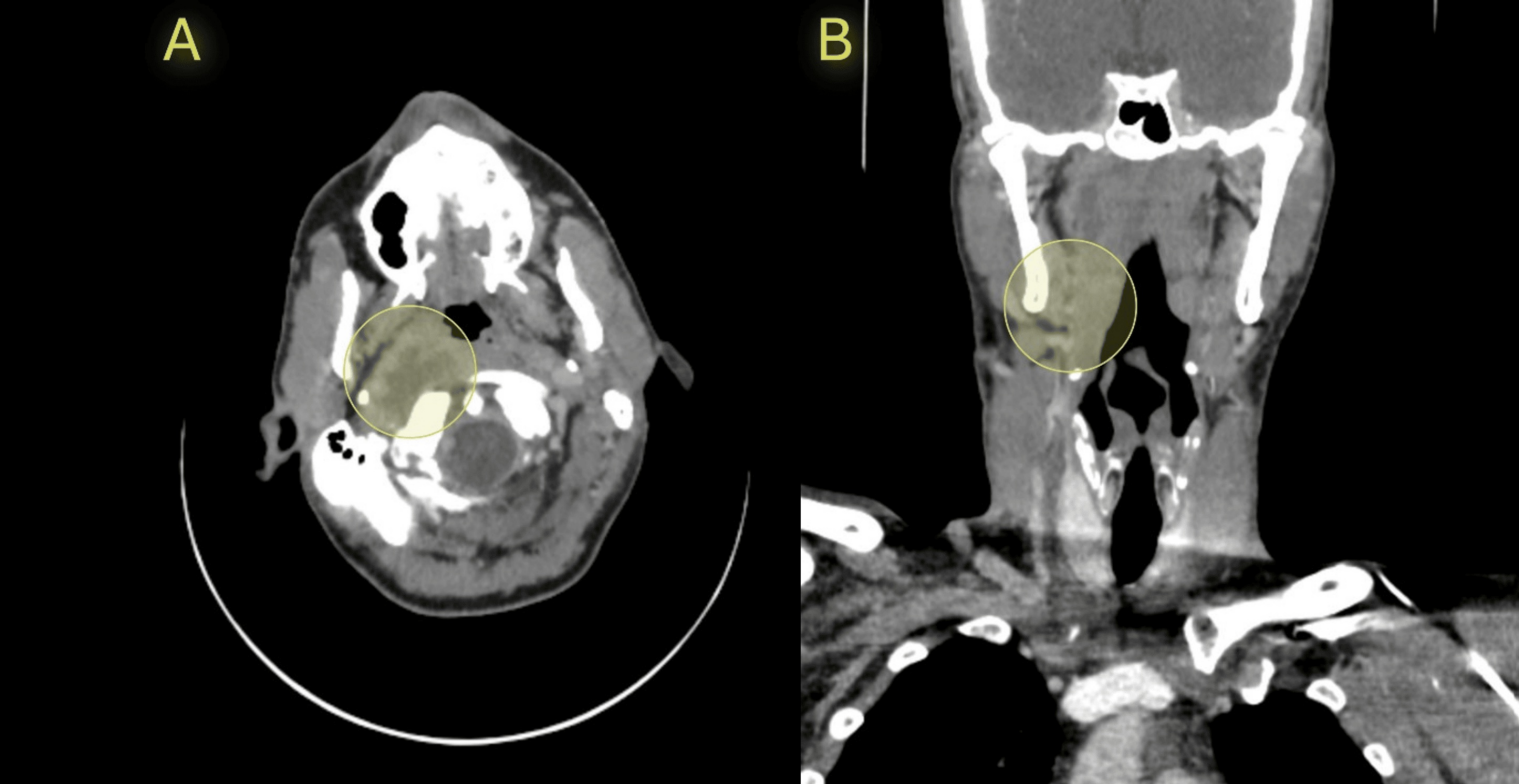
CT scan showing a large right parapharyngeal abscess (A) Axial view. Significant right parapharyngeal abscess located adjacent to the anterior border of the sternocleidomastoid muscle. (B) Coronal view.
